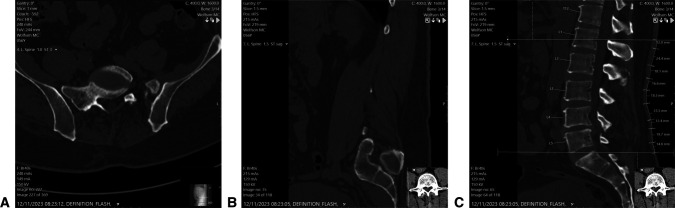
Radiographs demonstrating Wolfson modified Schober test measurements presented on CT. A, Radiograph demonstrating the PSIS landmark on CT axial and sagittal views. B, Radiograph demonstrating the PSIS caudal starting point. The PSIS tip was measured by marking the most prominent point of the PSIS, as measured by using axial and sagittal CT views, representing the PSIS as palpated on physical examination (marked arrow). On that level, scrolling back to a midline sagittal plane, a parallel line was drawn to the skin surface as described previously. C, Radiograph demonstrating WMST complete CT measurement. PSIS = posterior superior iliac spine, WMST = Wolfson modified Schober test.
